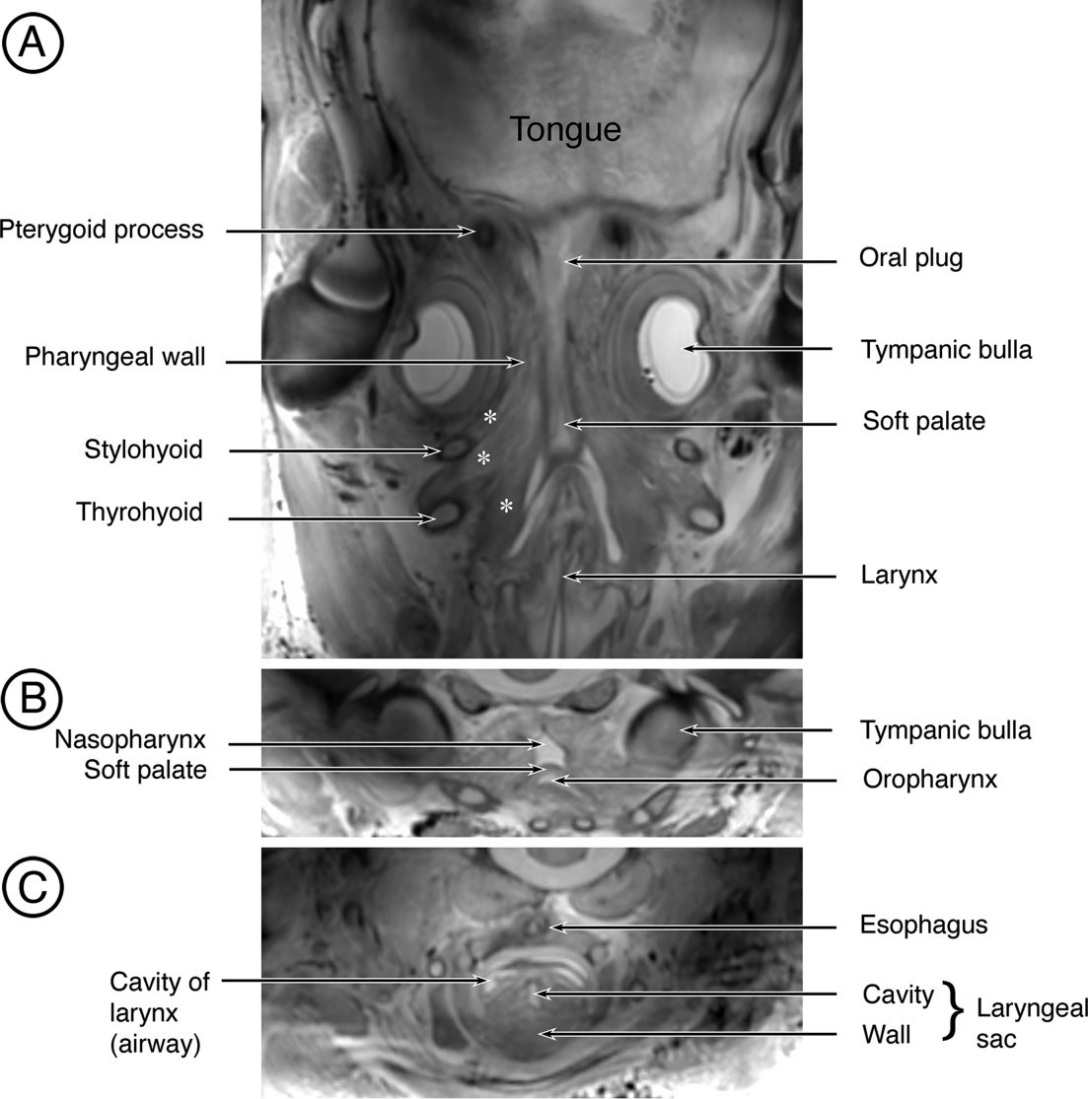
MRI scans of the soft palate and pharynx in a fetal fin whale. (A) MRI scan in the dorsal plane through the pharynx and soft palate showing the relationship of the soft palate and pharyngeal wall to the pterygoid processes and larynx. Muscles (middle and inferior constrictors) of the pharyngeal wall attaching to the hyoid apparatus and larynx are indicated by the asterisks. (B) Transverse section through the oropharynx, nasopharynx, and soft palate at a level near the caudal end of the tympanic bullae. (C) Transverse section through the larynx below and the beginning of the esophagus above. Also visible in this image is the laryngeal sac.
